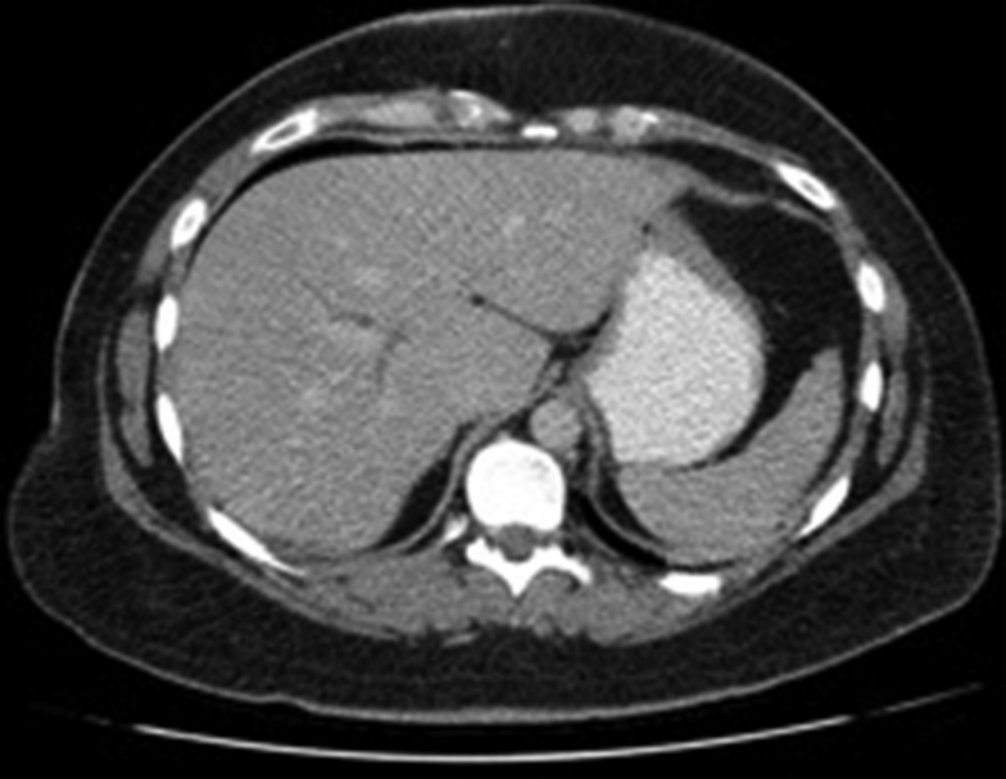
CT abdomen 5 years prior show no liver lesions.
